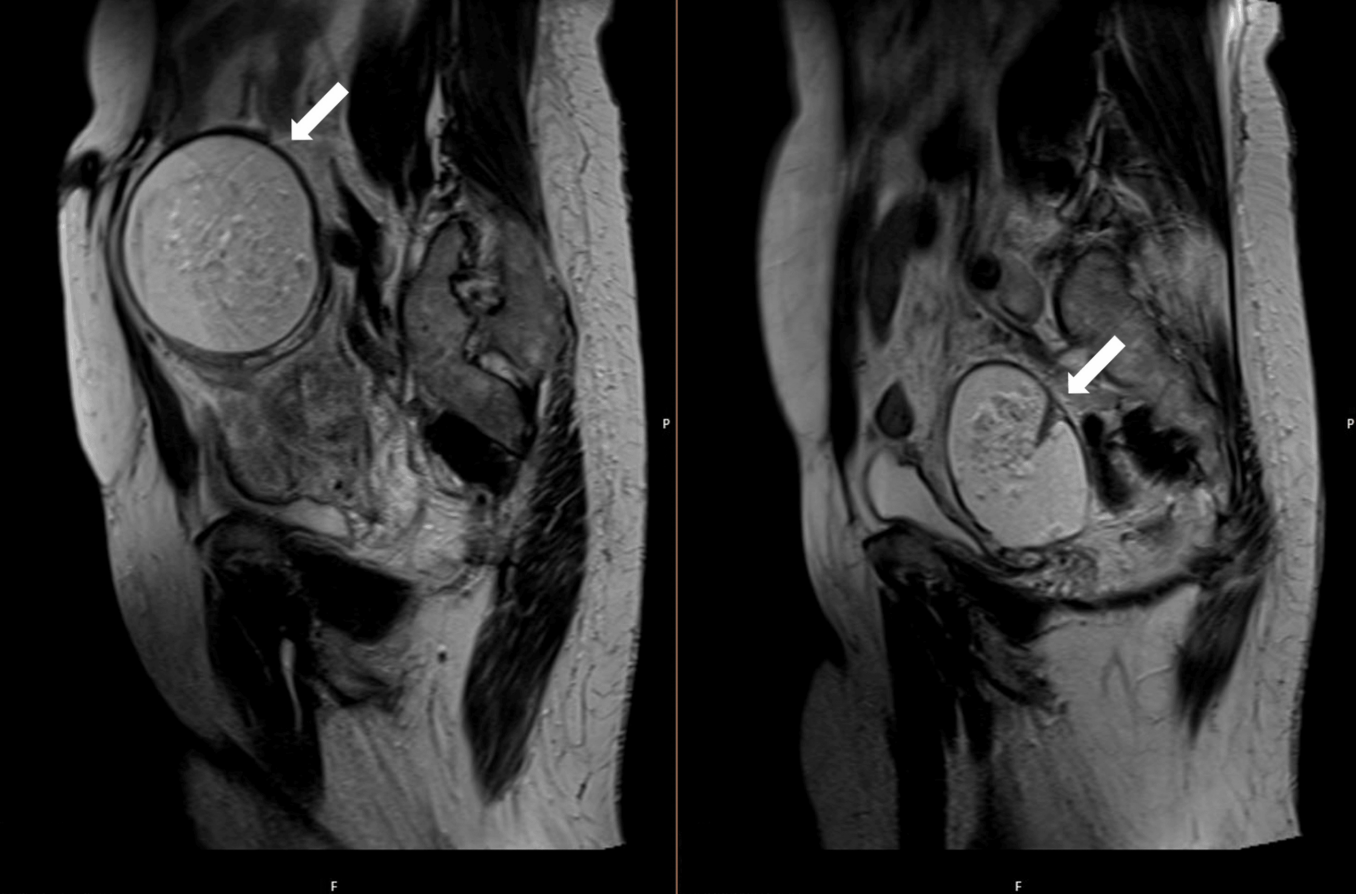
Sagital T2 MRI images of the pelvis showing bilateral adnexal masses (arrows) with predominant fatty components and internal nodular areas (hyperintense).
